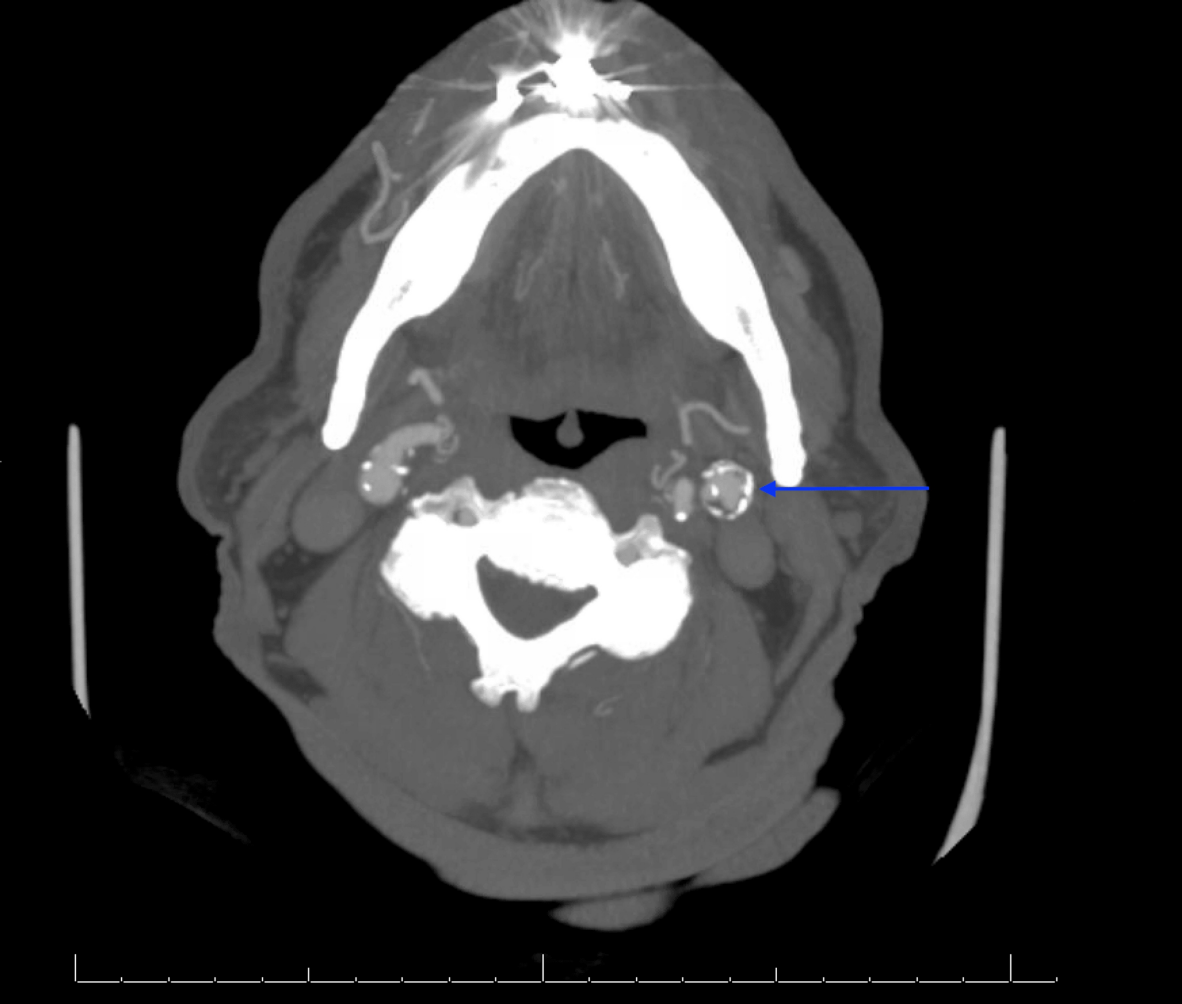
Axial CTA head/neck revealing an ulcerated plaque of the left cervical ICA origin (arrow) with 45-50% stenosis per NASCET criteria CTA: CT angiogram; ICA: internal carotid artery; NASCET: North American Symptomatic Carotid Endarterectomy Trial
